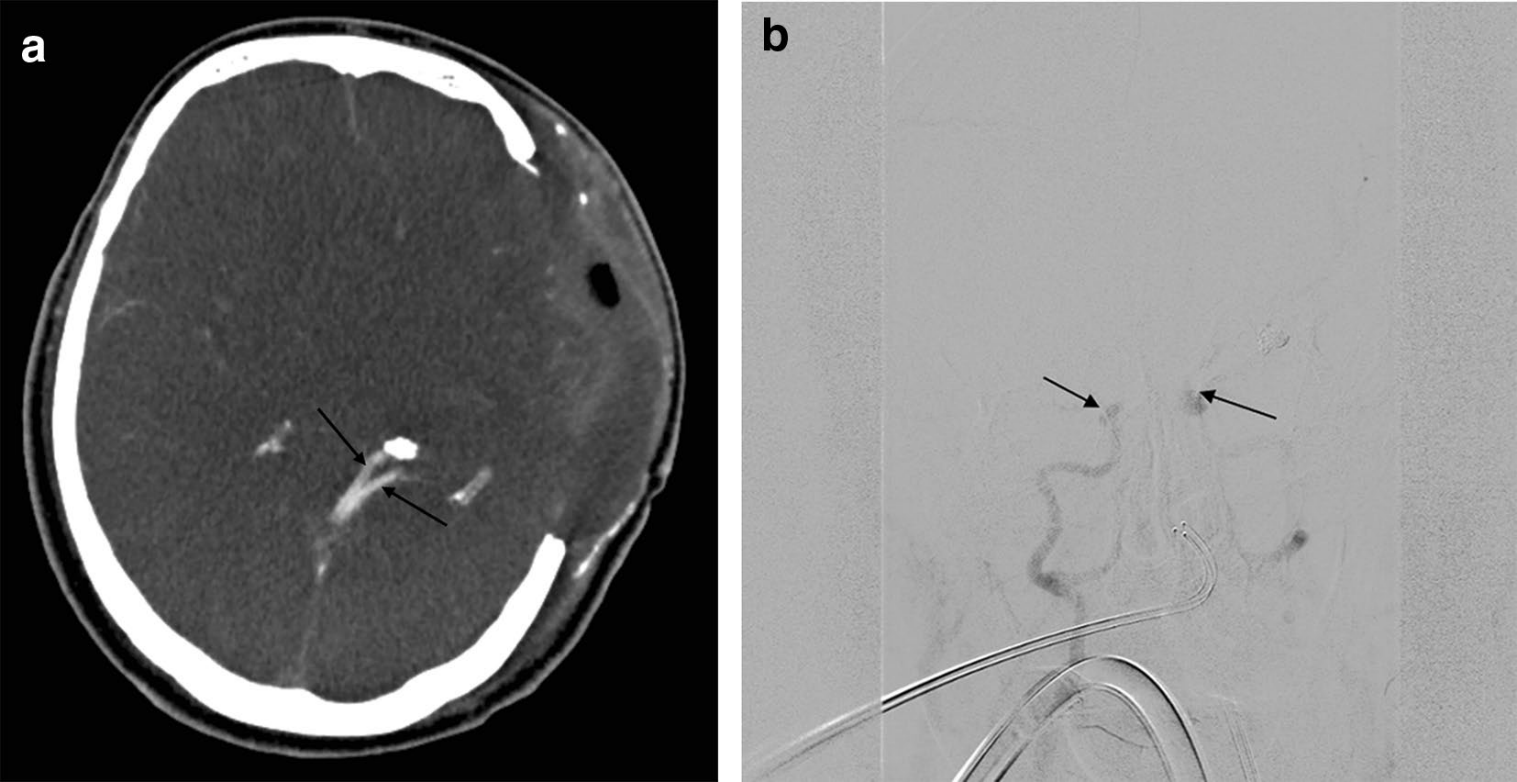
(a) Axial CTA (60 s phase), shows opacification of both internal cerebral veins (black arrows) in a craniectomy patient. (b) Digital Subtraction Angiography of the same patient in late phase (AP view), shows stasis filling on both cavernous ICAs (black arrows), with no venous outflow.
